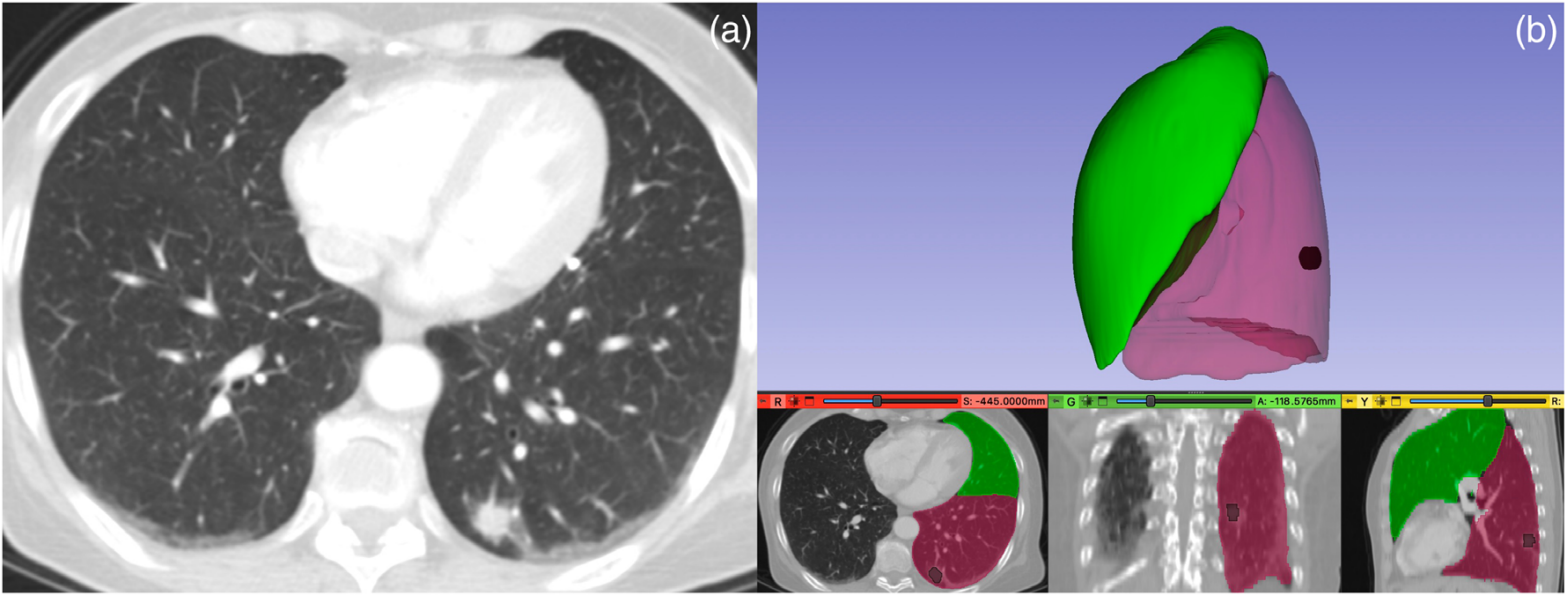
A 63‐year‐old woman with a lung adenocarcinoma. (a) Computed tomography (CT) scan showed a tumor located in the left lower lobe (LLL). (b) Digital 3D model with left upper lobe (LUL) (green), left lower lobe (LLL) in transparency (red) and nodule (black) in LLL. The surgical operation is shown in Video S2.
